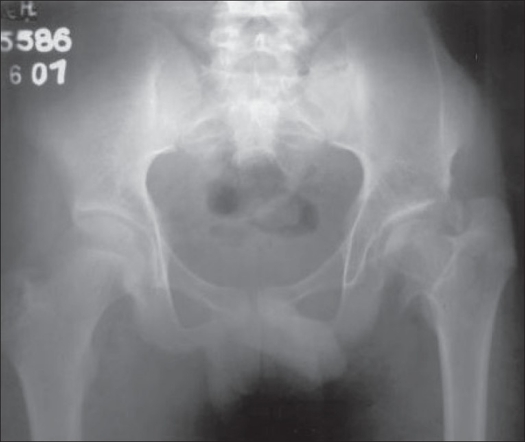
X-ray after six years of surgery shows near completely formed head
